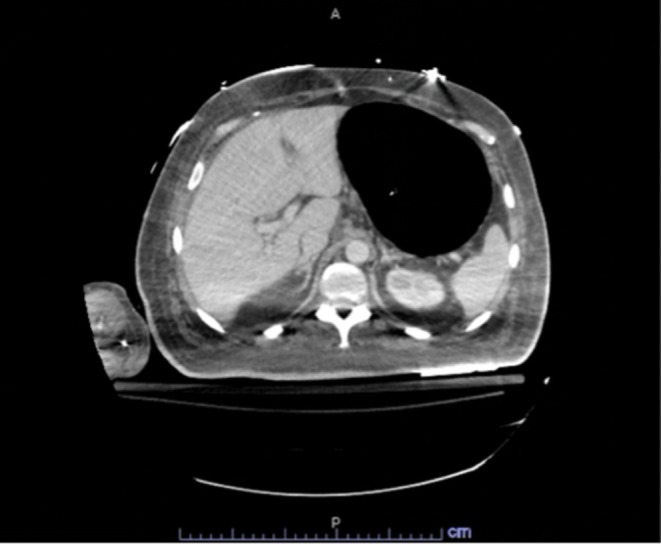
Computed tomography (CT) scan of the abdomen showing marked gaseous distension of the stomach.
